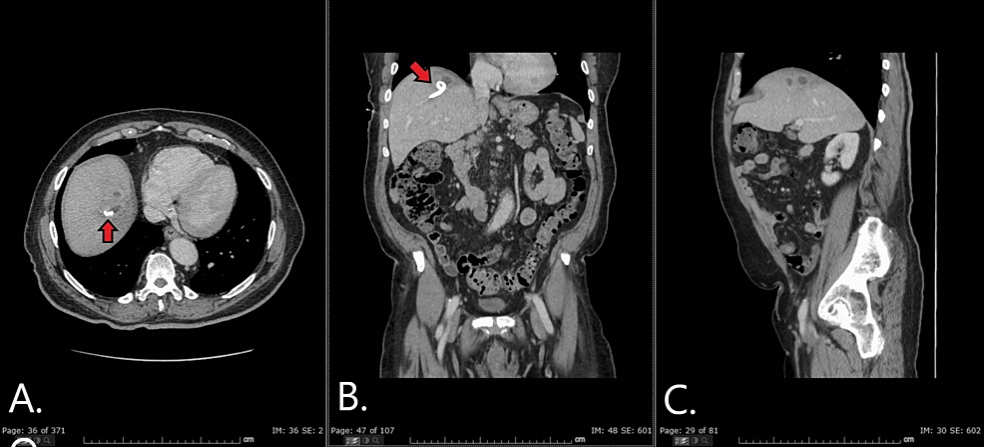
Axial (A), coronal (B), and sagittal (C) views of CT scan abdomen/pelvis with contrast three weeks post-discharge The images show a drastic decrease in abscess size post hepatic drain (red arrow) placement.
